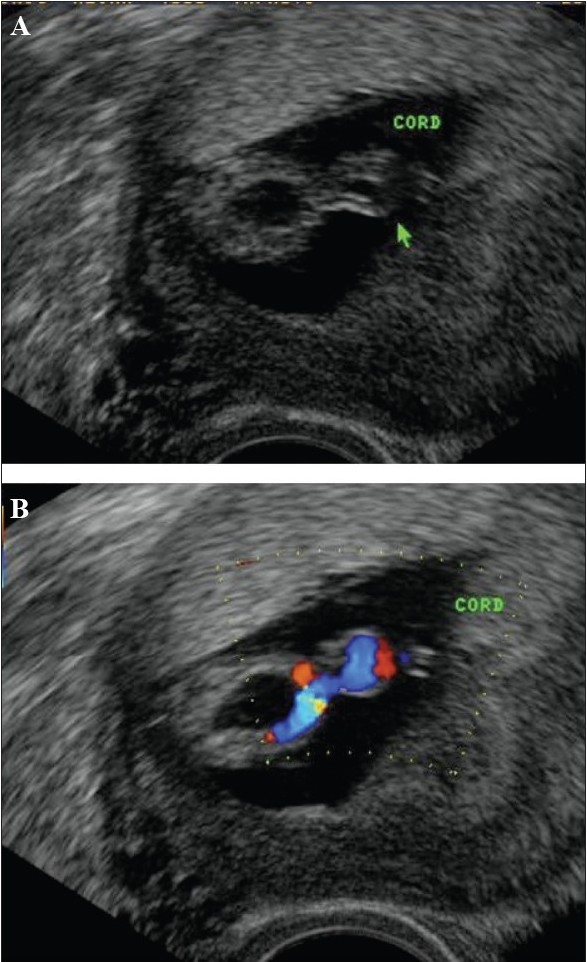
Grey-scale (A) and color Doppler (B) images of the cord suggest the presence a thick cord (arrow). The fetus had megacystis. Follow-up after a week revealed a cystic hygroma around the fetal skull; IUD occurred 1 week later
